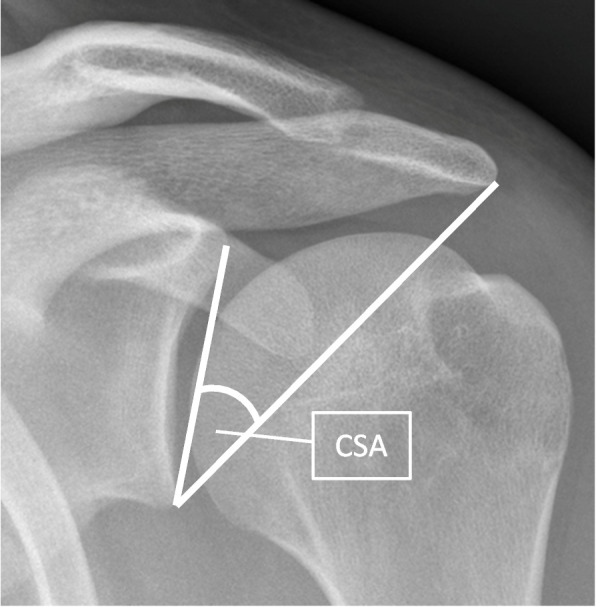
Measurement of the critical shoulder angle using an anteroposterior radiograph of the shoulder. The angle is between a line connecting the inferior with the superior border of the glenoid fossa and another connecting the inferior border of the glenoid with the most inferolateral point of the acromion
